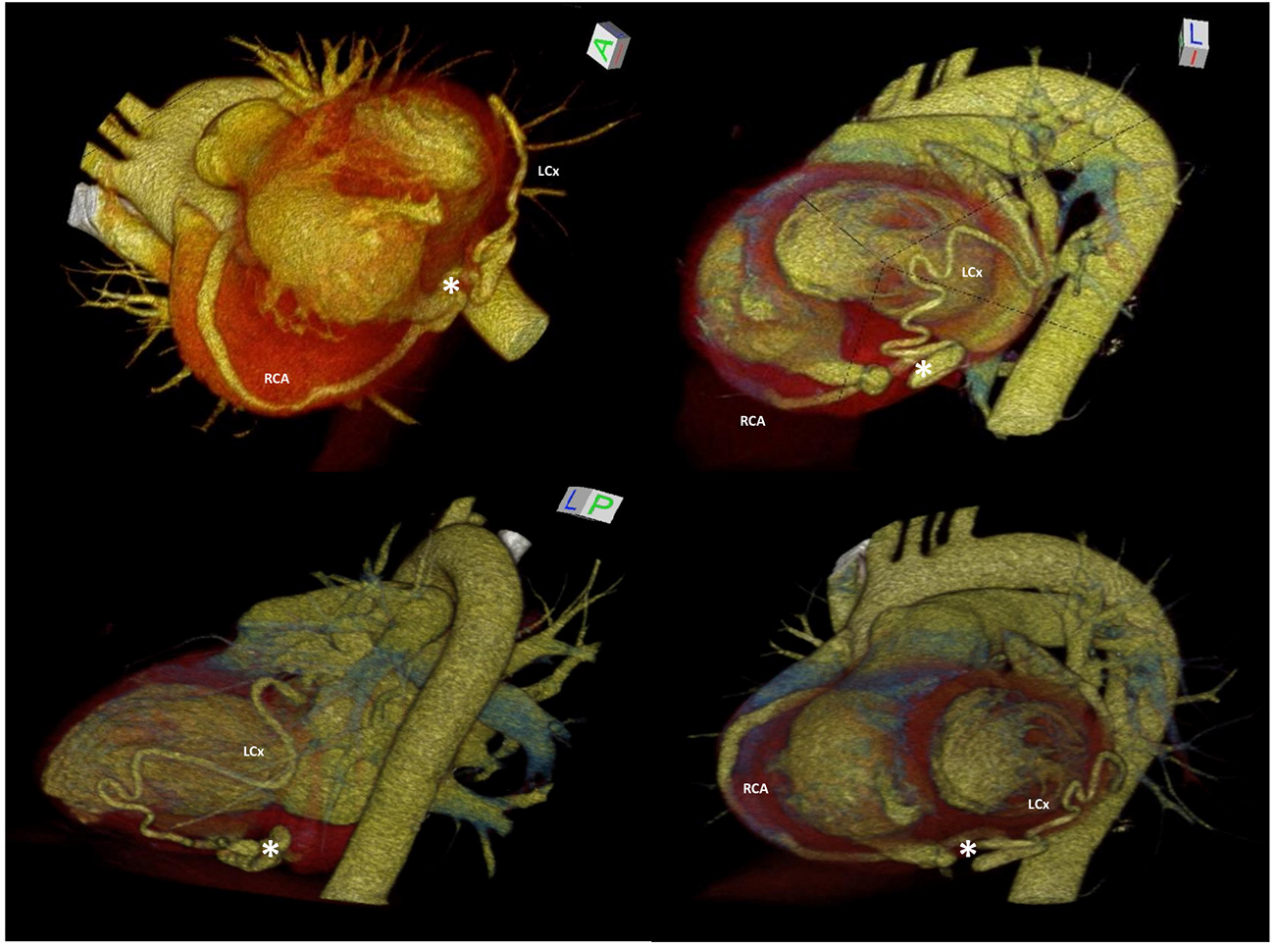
3D-MDCTA volume-rendering reconstruction showing dilated and tortuous right coronary artery and left circumflex artery forming at the base of the heart a distal anastomosis (white asterisk), followed by drainage into the right ventricle through the posterior-lateral wall. 3D-MDCTA, three-dimensional multidetector computed tomography angiography; LM, left main coronary artery; RCA, right coronary artery; LAD, left anterior descending; LCx, left circumflex.
